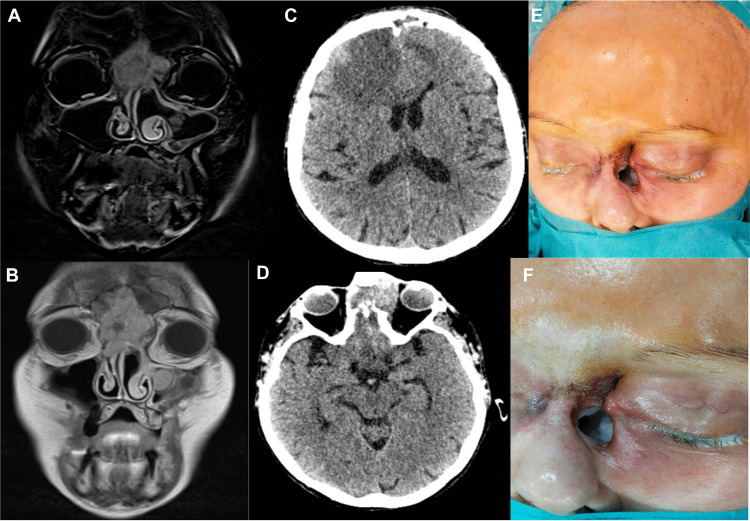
Preoperative MRI and CT of the head and clinical presentation. (A) Coronal MRI demonstrating a frontal mass extending inferiorly into the nasal cavity. (B) Axial CT showing a frontal mass with hyperdense margins. (C) Axial bone-window CT showing destructive changes of the nasal bones. (D) Sagittal bone-window CT demonstrating destruction of the cribriform plate and intracranial pneumocephalus. (E) Invasive tumor with nasal bone erosion and infiltration of the nasal root skin. (F) Direct communication between the nasal cavity and external environment after previous reconstruction failure
